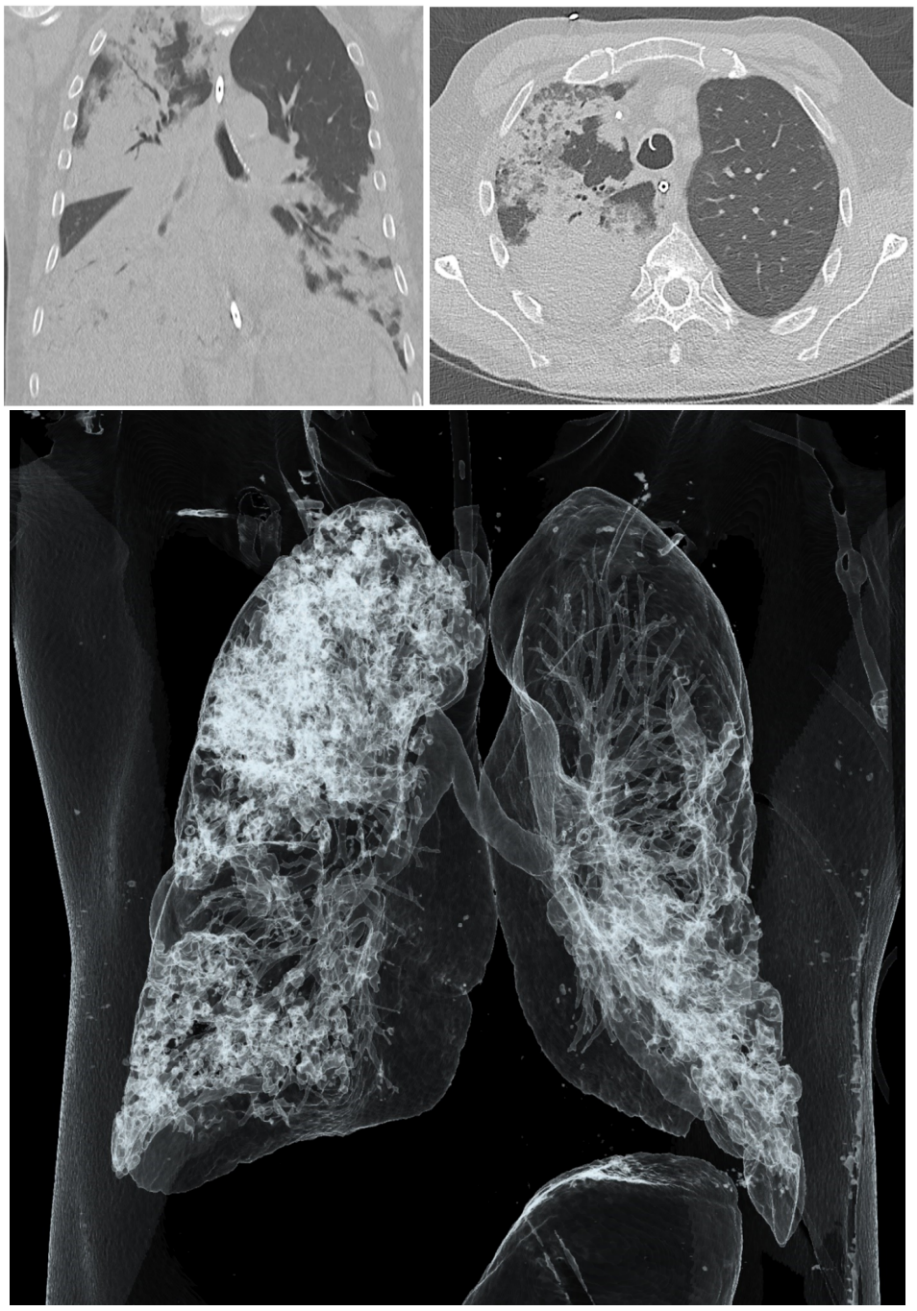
CT imaging of patient’s chest at hospital admission. Upper left panel: Axial non-contrast CT image showing infiltrates in the upper and lower lobes of the right lung. Upper right panel: Coronal non-contrast CT image demonstrating infiltrates in the upper right and both lower lobes of the lung. Lower panel: 3D reconstruction of the CT showing the relative sparing of the left upper lobe.
